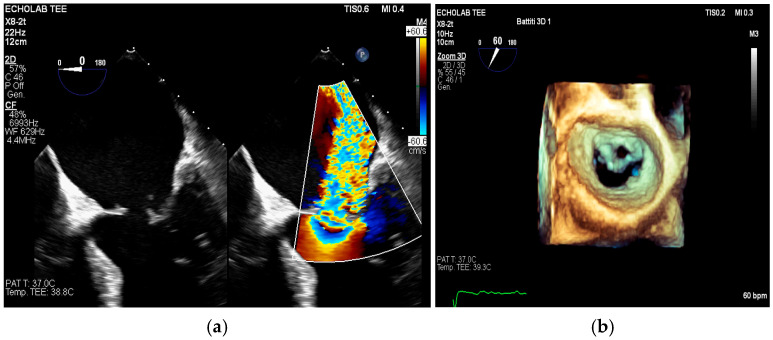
(a,b): On the left, leaflet perforation is suspected from the Color Doppler pattern observed (a). On the right (b), the 3D zoom further defines the origin of the perforation. This is an original, de-identified clinical image from our institution. Patient consent for publication was obtained.
